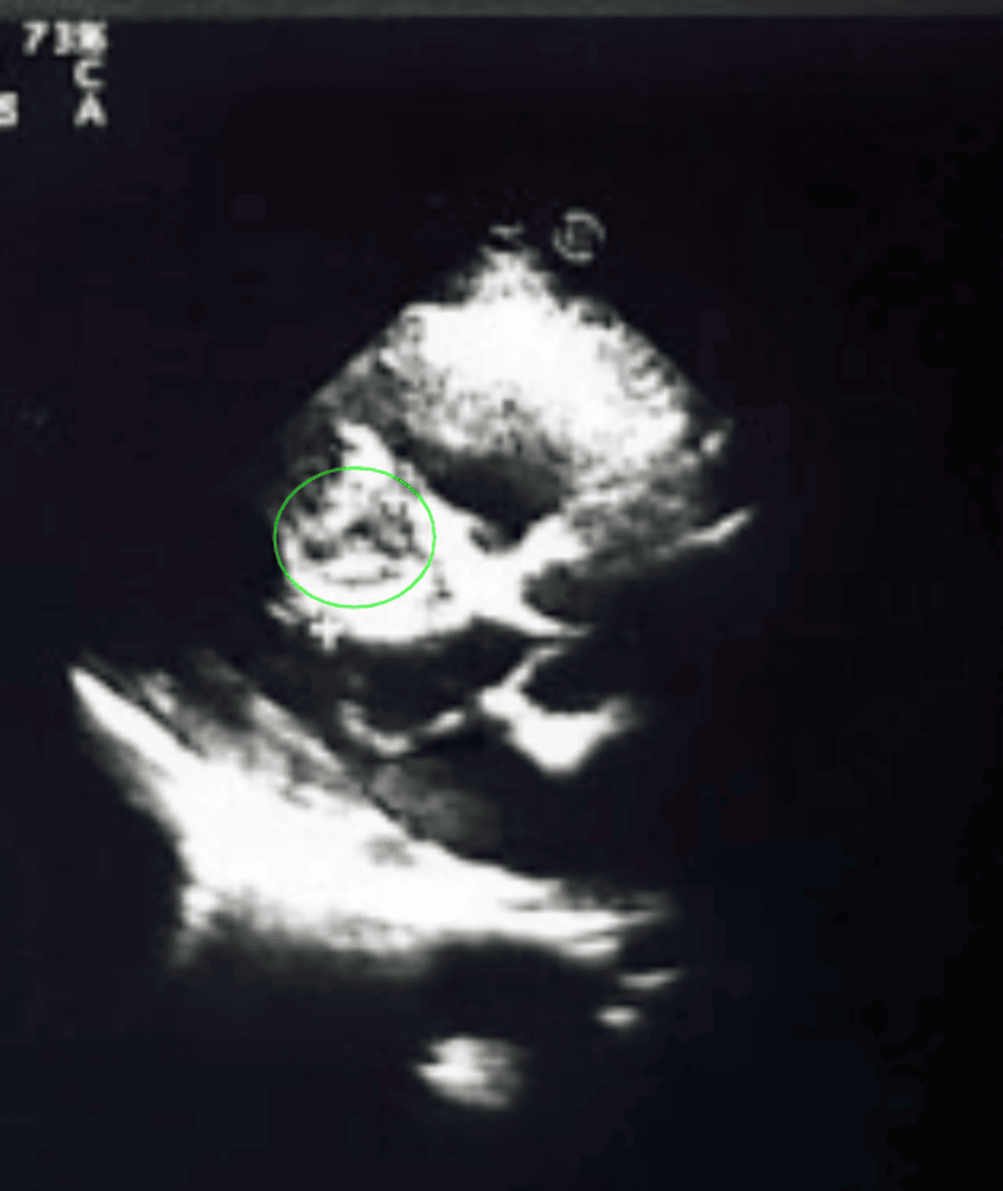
Transthoracic echocardiogram showing a cystic mass at basal interventricular septum.
